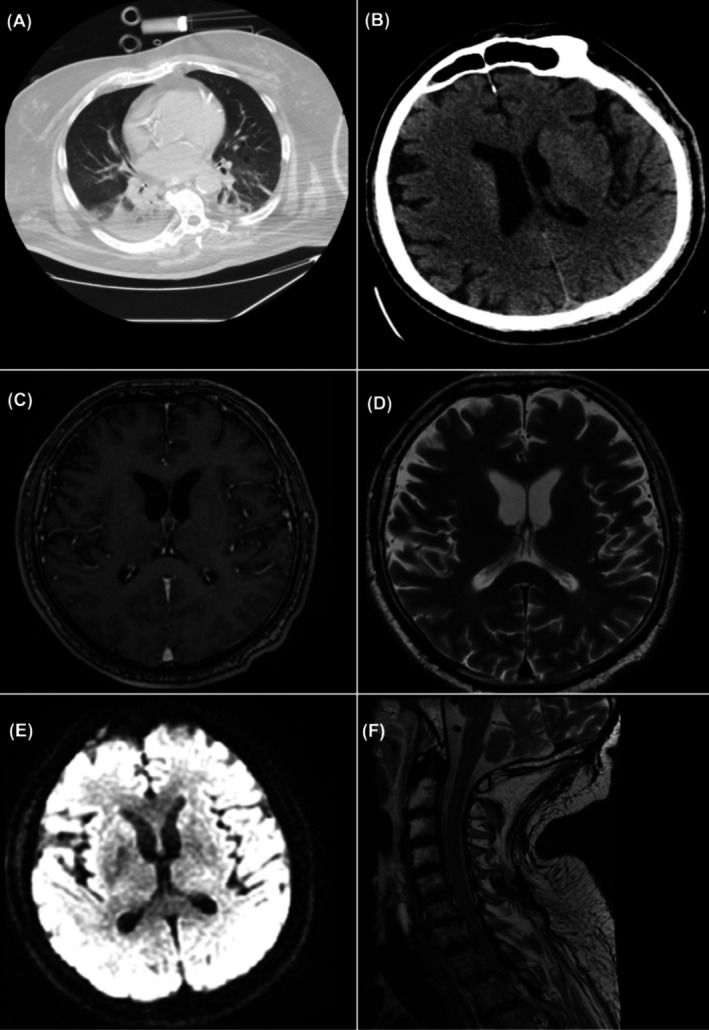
Cerebral and spinal magnetic resonance imaging. Note no pathological intracranial or spinal findings. (A) Chest CT (Axial, Lung Window): Patchy ground‐glass opacities predominantly in the lower lobes, more pronounced posteriorly, associated with bilateral atelectasis. Findings suggestive of aspiration pneumonia. (B) Non‐Contrast Head CT (Axial): Lateral ventricles displaying moderate cortical atrophy, but no signs of ischemia or space‐occupying lesions. (C) Brain MRI—T1‐weighted Post‐Contrast (Axial): No abnormal contrast enhancement. No signs of ring‐enhancing lesions or abnormal meningeal enhancement. No evidence of abscess, neoplasm, or active inflammation. (D) Brain MRI—T2‐weighted (Axial): No signs of encephalopathy or other significant pathological findings. (E) Brain MRI—Diffusion‐Weighted Imaging (DWI): No signs of myelopathy or other significant pathological findings. (F) Cervical Spine MRI—T2‐weighted Sagittal: Normal cervical alignment. No evidence of spinal cord compression, demyelination, or edema. The spinal cord shows normal signal intensity. No herniated discs or spinal canal stenosis visible in this slice. No MRI evidence of cervical myelopathy or demyelinating disease.
